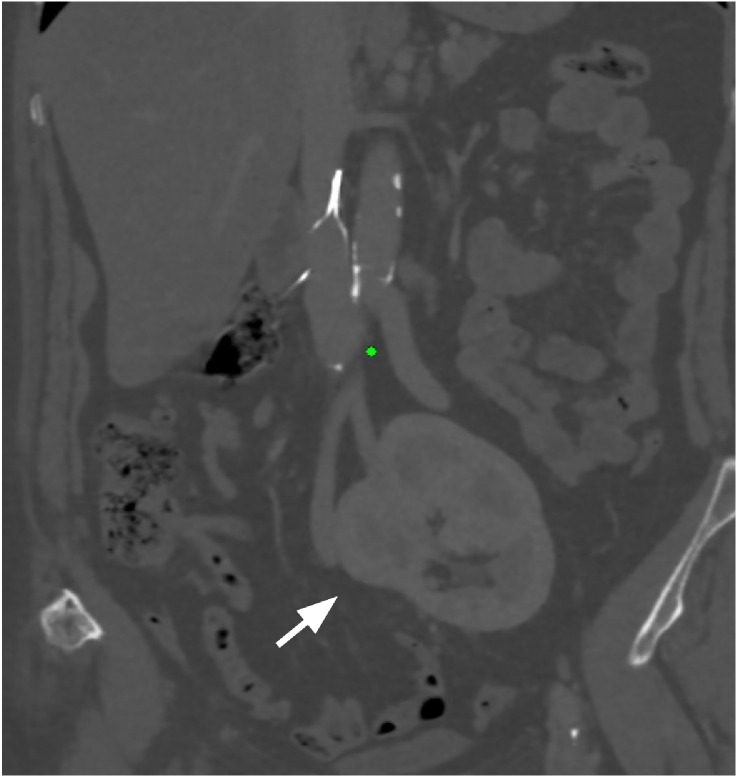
Coronal non-contrast CT kidney–ureter–bladder image demonstrating an incidental fused ectopic pelvic kidney without obstruction.
